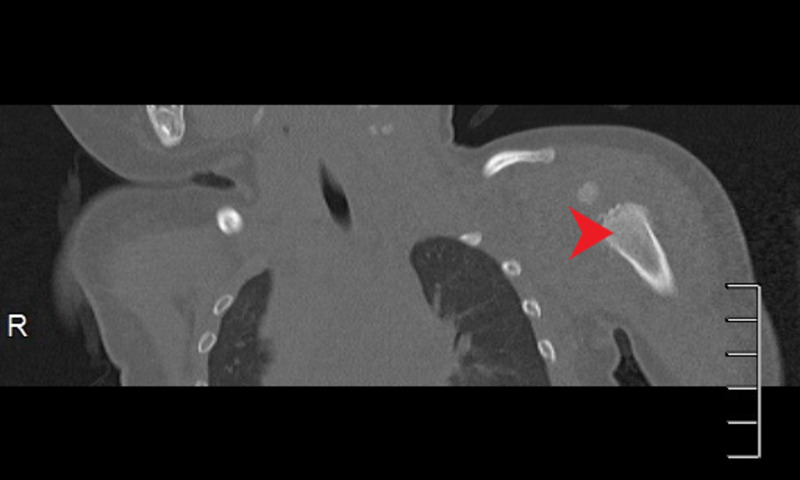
CT scan showing periosteal reaction in metaphysis of left humerus.
